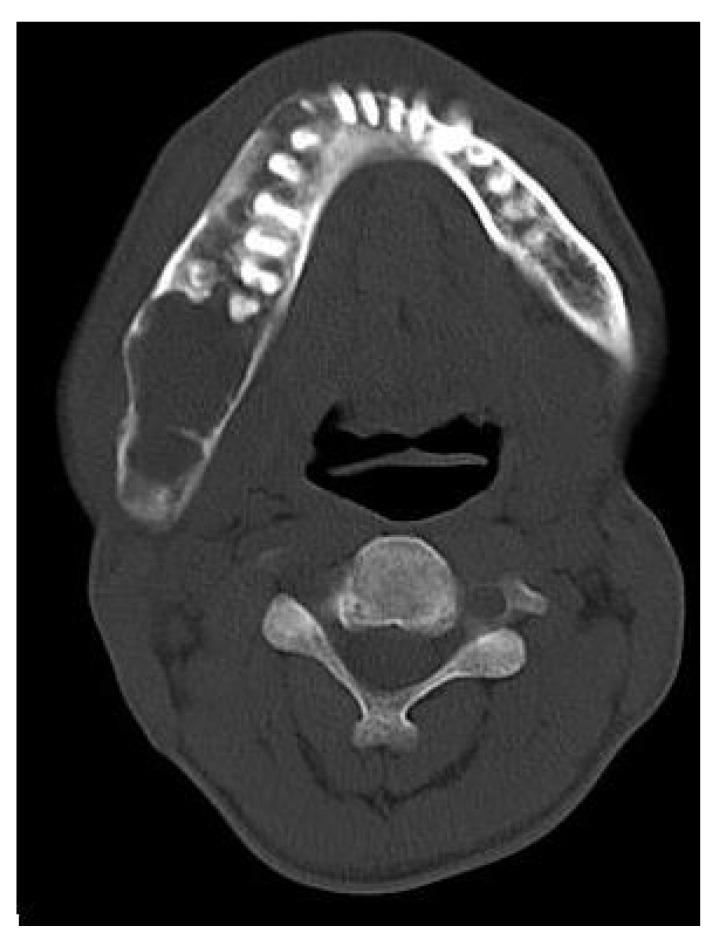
Axial CT section with evidence of a cystic lesion in the right mandible extending from body of the mandible to the ramus of right side.
